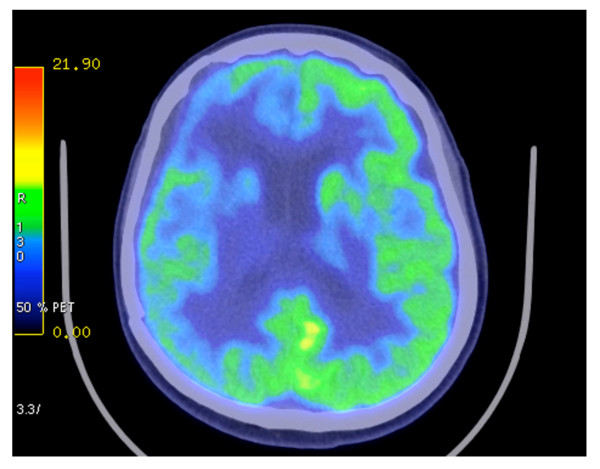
Positron emission tomography with fluoro-deoxy-glucose (FDG-PET) imaging  showing no evidence of recurrent tumor.
